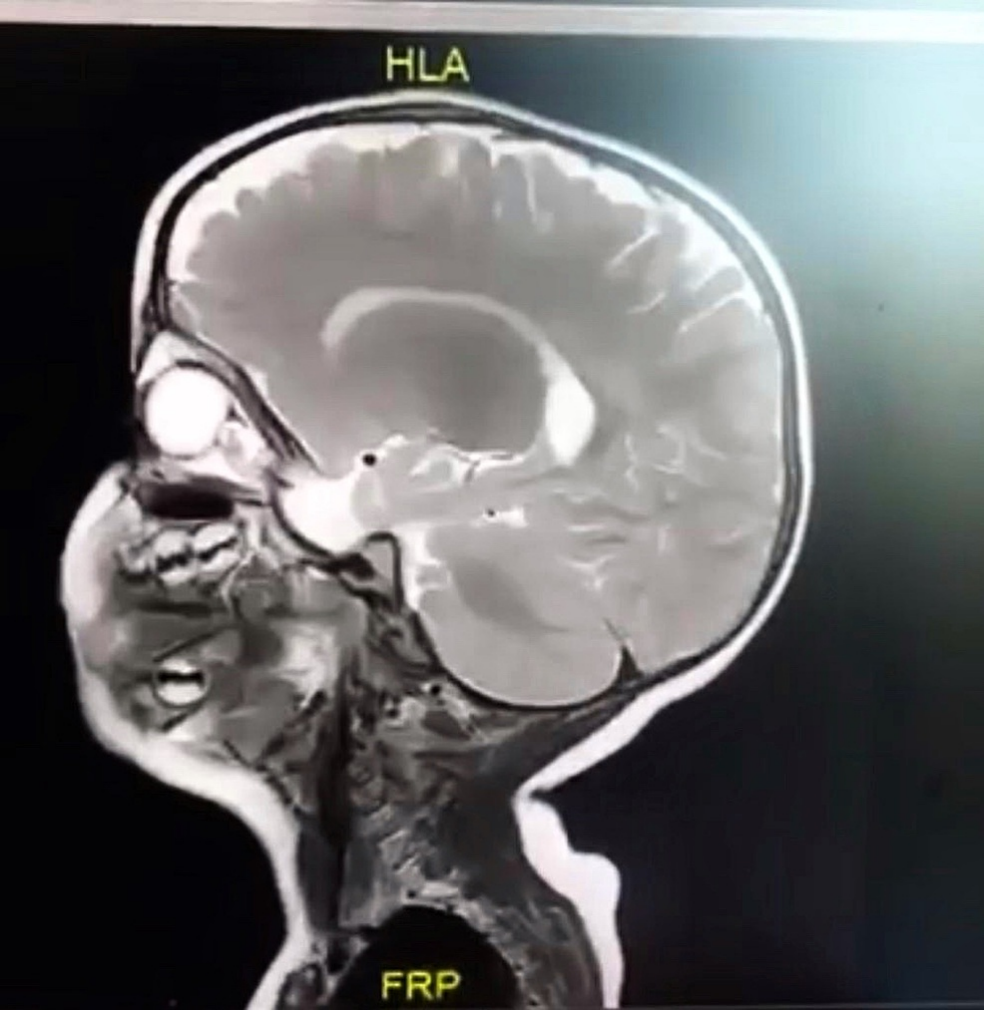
MRI of the face showing the nasal dorsum lesion measuring 10 × 16 × 10 mm with draining sinus tract anterior to the nasofrontal junction with no intracranial extension. MRI: magnetic resonance imaging
